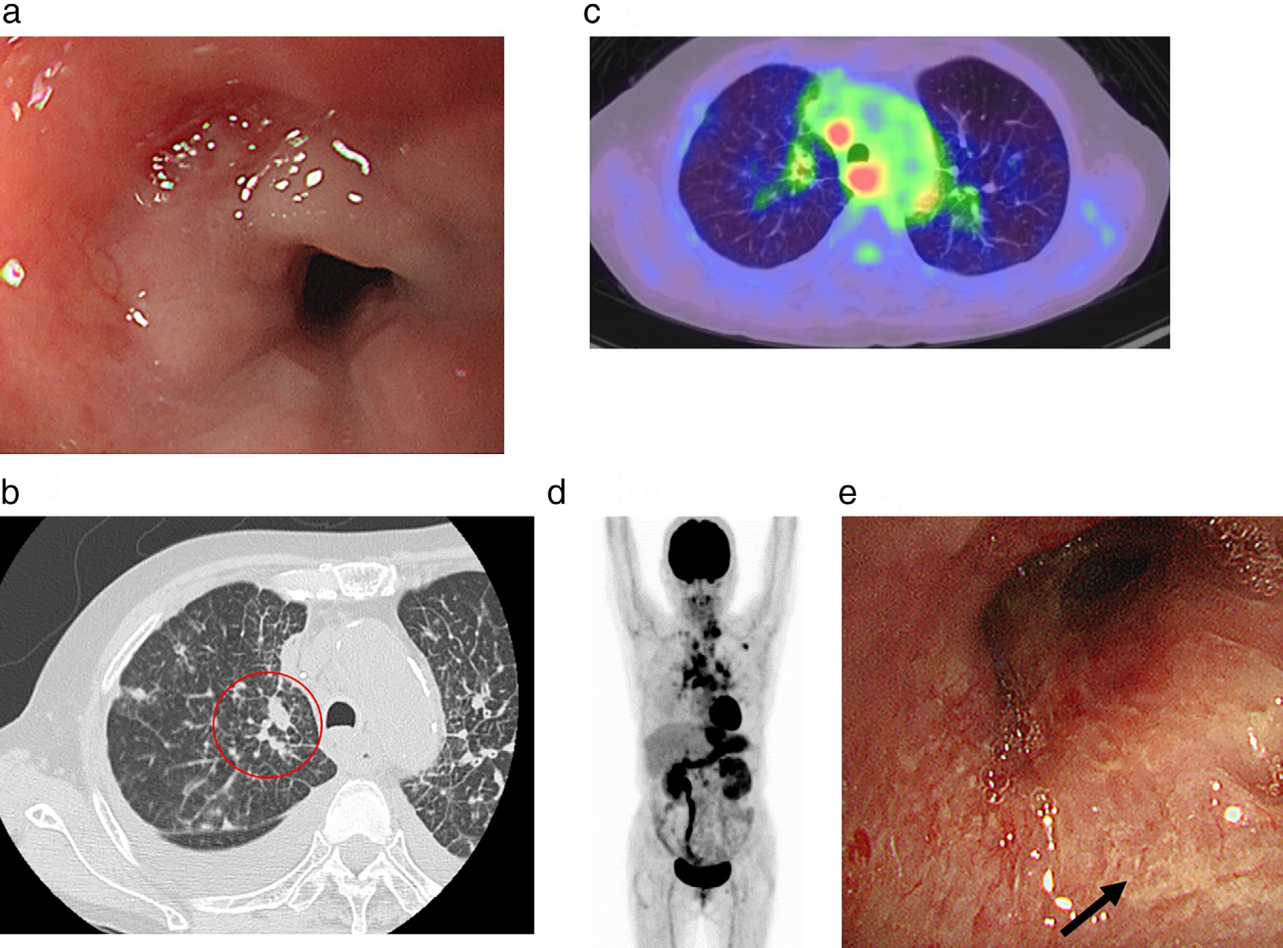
(a) Upper gastrointestinal endoscopy revealed an esophageal stricture. (b) Computed tomography (CT) showed bilateral cancerous lymphangiopathy and a 5 mm nodule in the right lung. (c,d) Positron emission tomography (PET)‐CT showed high accumulation in the lung nodule, thyroid, and cervical, mediastinal, and intraperitoneal lymph nodes. (e) Bronchoscopy showed the mucous membrane of the right upper lobe branch had white mucosal irregularities
